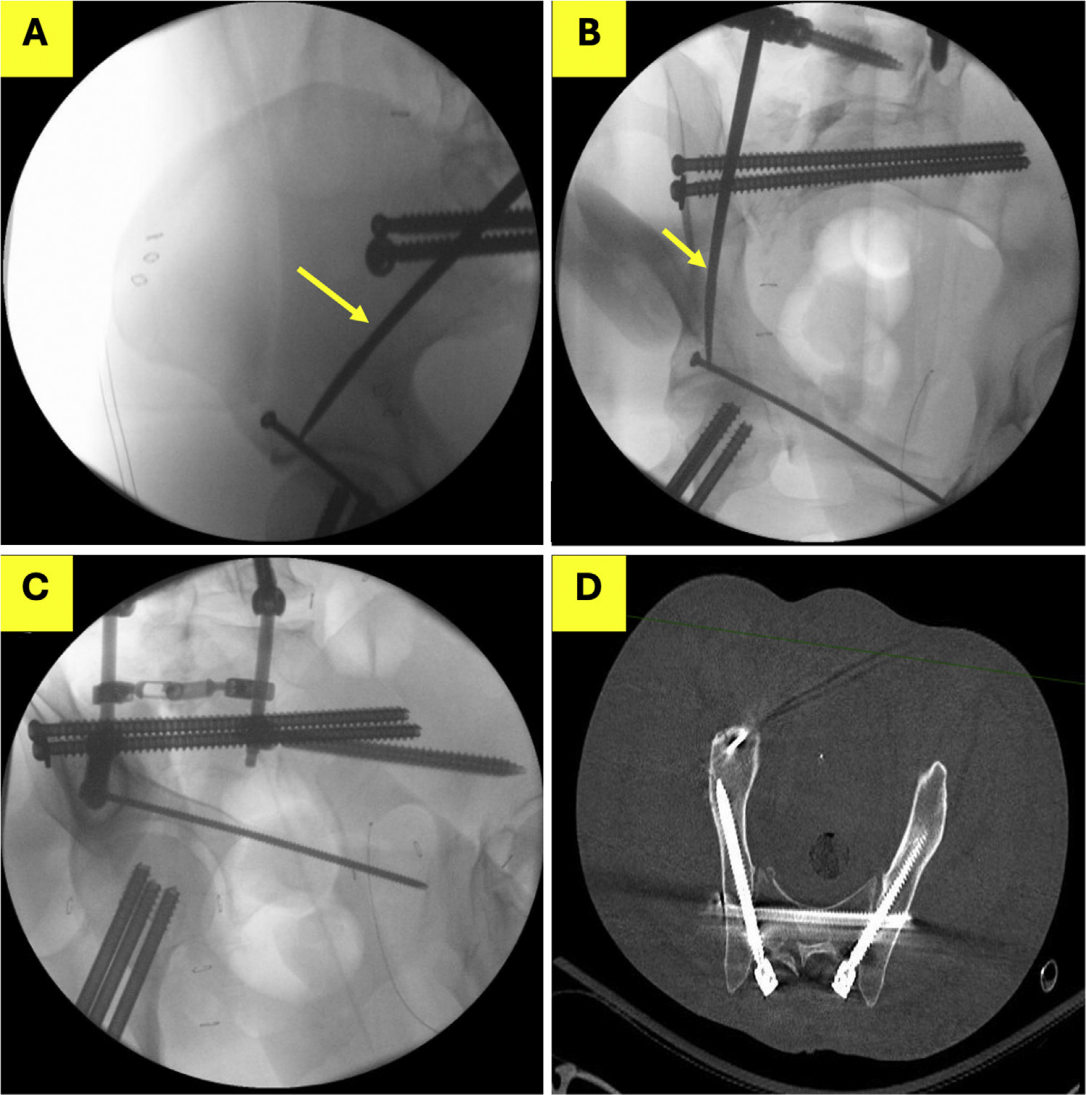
A 42-year-old patient undergoing percutaneous lumbopelvic fixation for a complex pelvic ring fracture. (A) Iliac oblique view guiding the cranial/caudal trajectory of the pedicle finder (yellow arrow). The pedicle finder is cranial to the sciatic notch and directed towards the distal aspect of the anterior inferior iliac spine. (B) Obturator inlet view guiding the medial-lateral trajectory of the pedicle finder. (C) The obturator outlet (“teardrop”) view is used as a confirmatory view after placement of the right iliac screw. (D) Postoperative CT demonstrates safe intraosseus placement of the pelvic screw occupying the length of the corridor.
